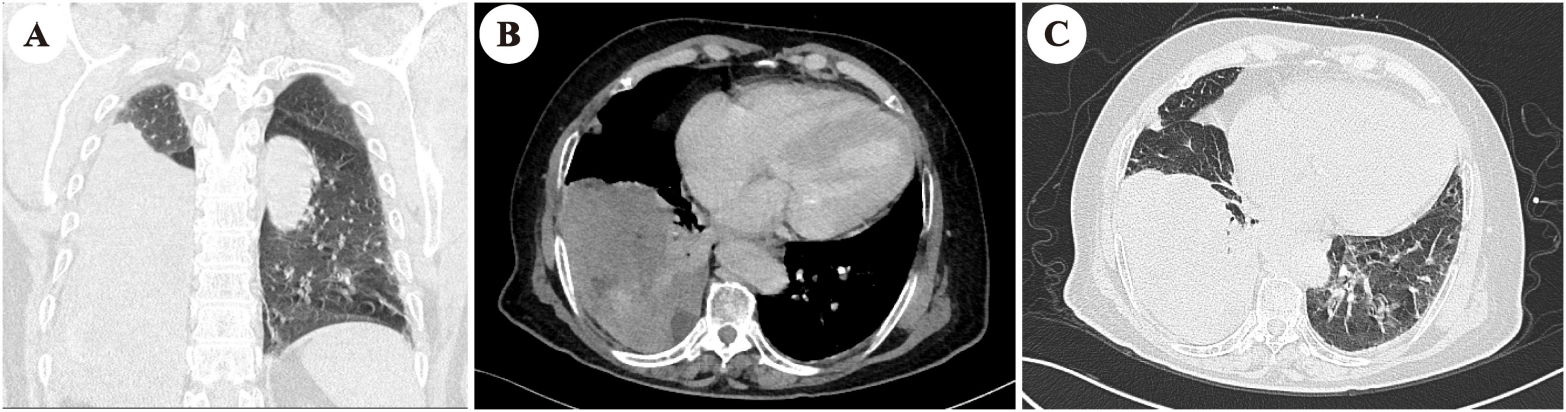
The chest CT revealed a giant heterogeneous mass in the right lower thoracic cavity (13 cm × 8.6 cm). (A) Coronal, maximum intensity projection CT images of the chest. (B) Sagittal portal phase imaging. (C) Sagittal arterial phase images.
